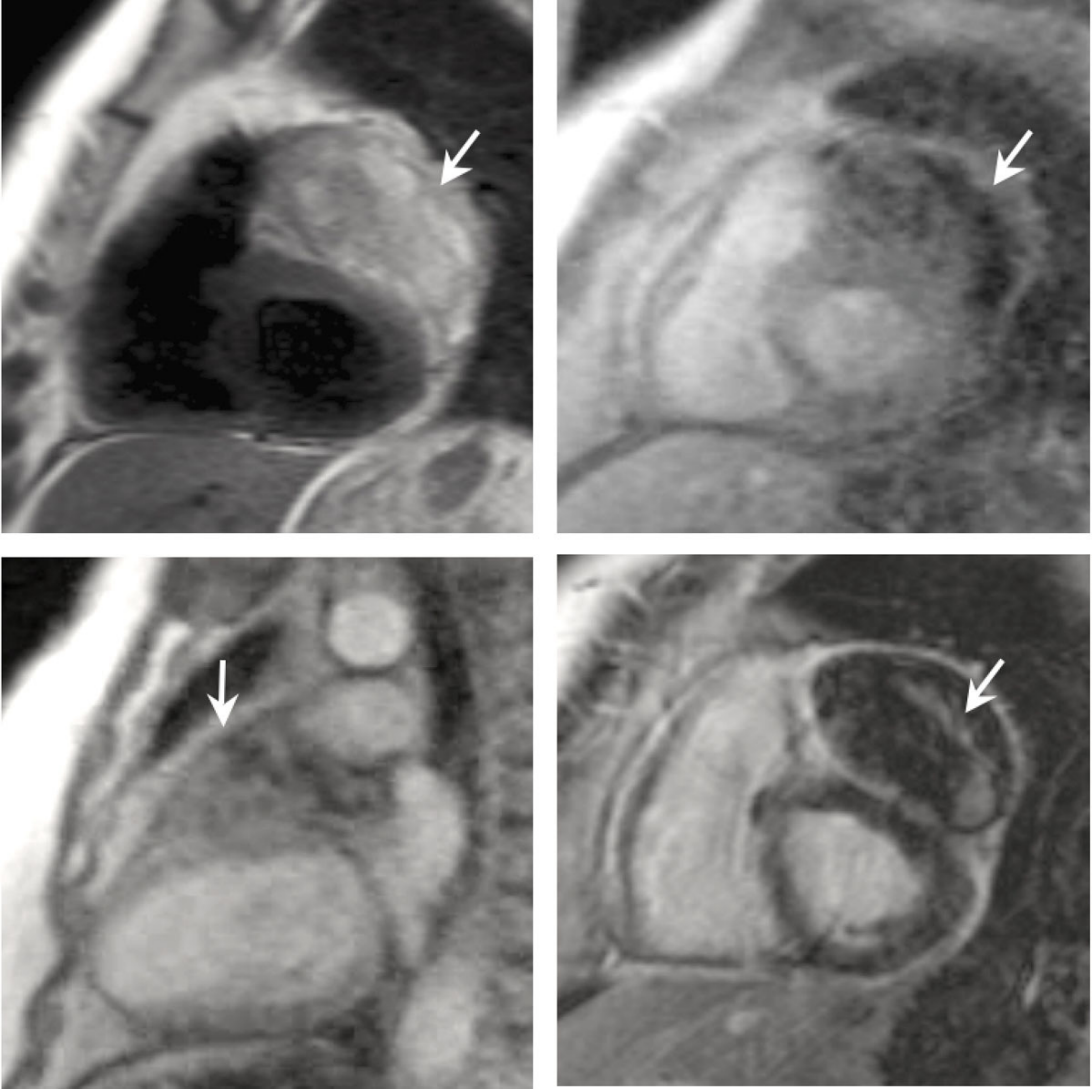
Patient with metastatic undifferentiated sarcoma to the heart (arrow) undergoing CMR imaging. T2-weighted imaging (top left) shows varying signal intensity with more edema in the periphery. Resting perfusion imaging (top right: short axis view, bottom left: long axis view) shows a gradient perfusion with the peripheral portion of the mass having less vascularity and perfusion. Delayed enhancement imaging (bottom right) shows a gradient enhancement suggesting more necrosis along the outer portion of the mass.
